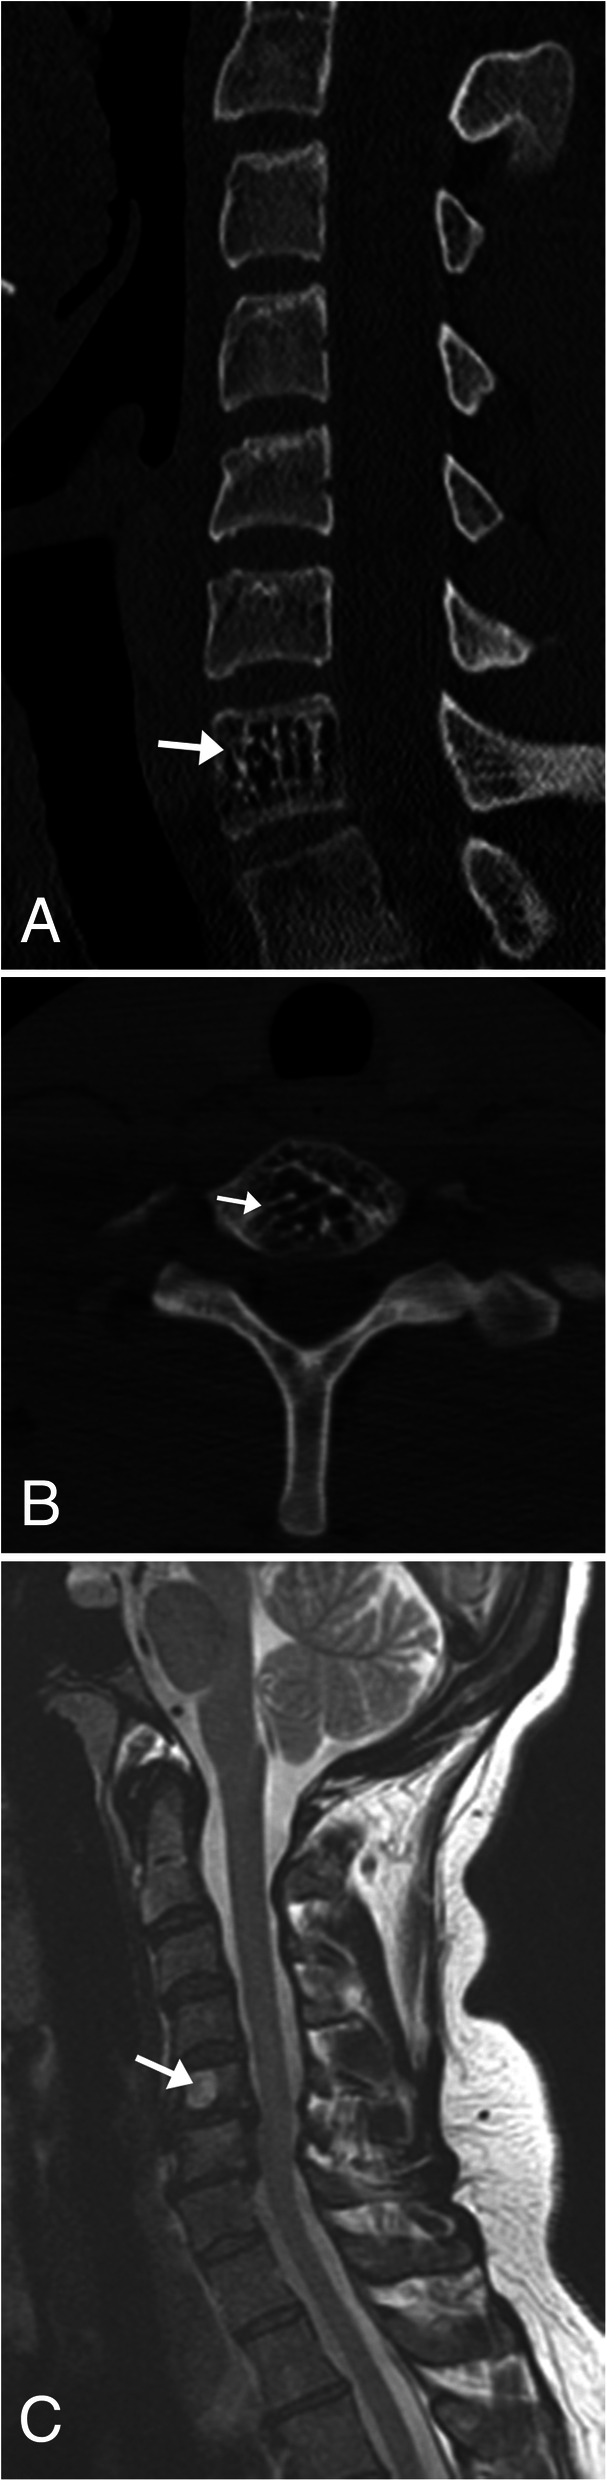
Intraosseous venous vascular malformation (formerly hemangioma). a Sagittal and (b) axial CT of the cervical spine showing the thickened vertical trabeculation (arrow) in a background of fatty tissue classically seen in the lesion at C7, described as the “corduroy sign” in the sagittal or coronal planes and a polka-dot appearance in the axial plane. c Sagittal T2WI MR image of the cervical spine in a companion case demonstrates the hyperintense nature of a lesion in the C5 vertebral body due to a fat component
